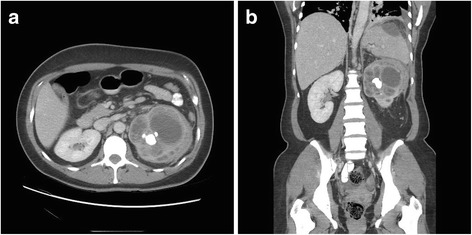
Computed tomography of the abdomen and pelvis demonstrating xanthogranulomatous pyelonephritis of the left kidney. a Axial section at the level of the renal calyces demonstrating markedly abnormal appearance of the left kidney. The renal pelvis is contracted around a staghorn calculus with surrounding distension and ballooning in the upper pole calyces, with surrounding soft tissue stranding and enlarged perinephric and retroperitoneal lymph nodes which are presumed to be reactive. b Coronal section of the abdomen and pelvis showing aforementioned changes in the left kidney with superior extension into the spleen parenchyma. Tracking of the inflammatory perinephric soft tissue along the posterior aspect of the spleen (not shown in this view) connects with a large hypodense collection under the splenic capsule, which also appears to communicate across left hemidiaphragm with resulting left-sided pleural effusion
